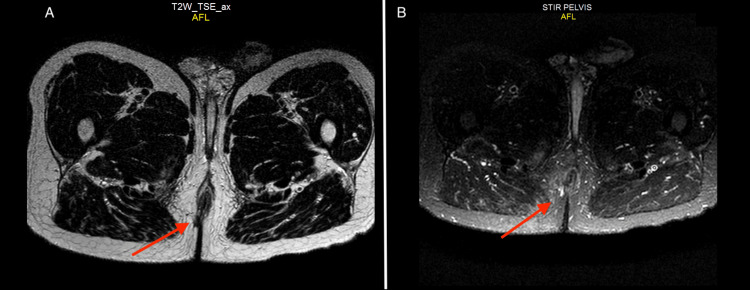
Post-treatment pelvic MRI (A) Axial section T2 sequence, (B) Axial section T2-STIR sequence demonstrating disappearance of the perianal process (red arrows), suggestive of complete tumor response. STIR: Short tau inversion recovery
